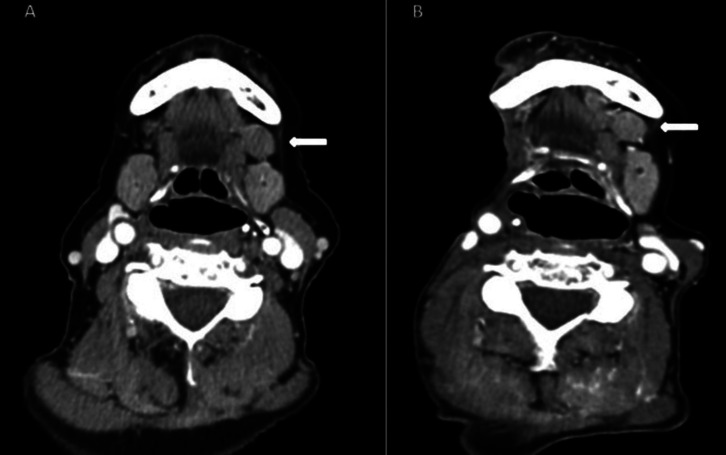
A) Axial cervical CT after administration of intravenous contrast (iv) in which a left accessory submaxillary gland is observed in front of the main gland. B) Cervical CT with same image on the left side two years after the right cervical emptying.
